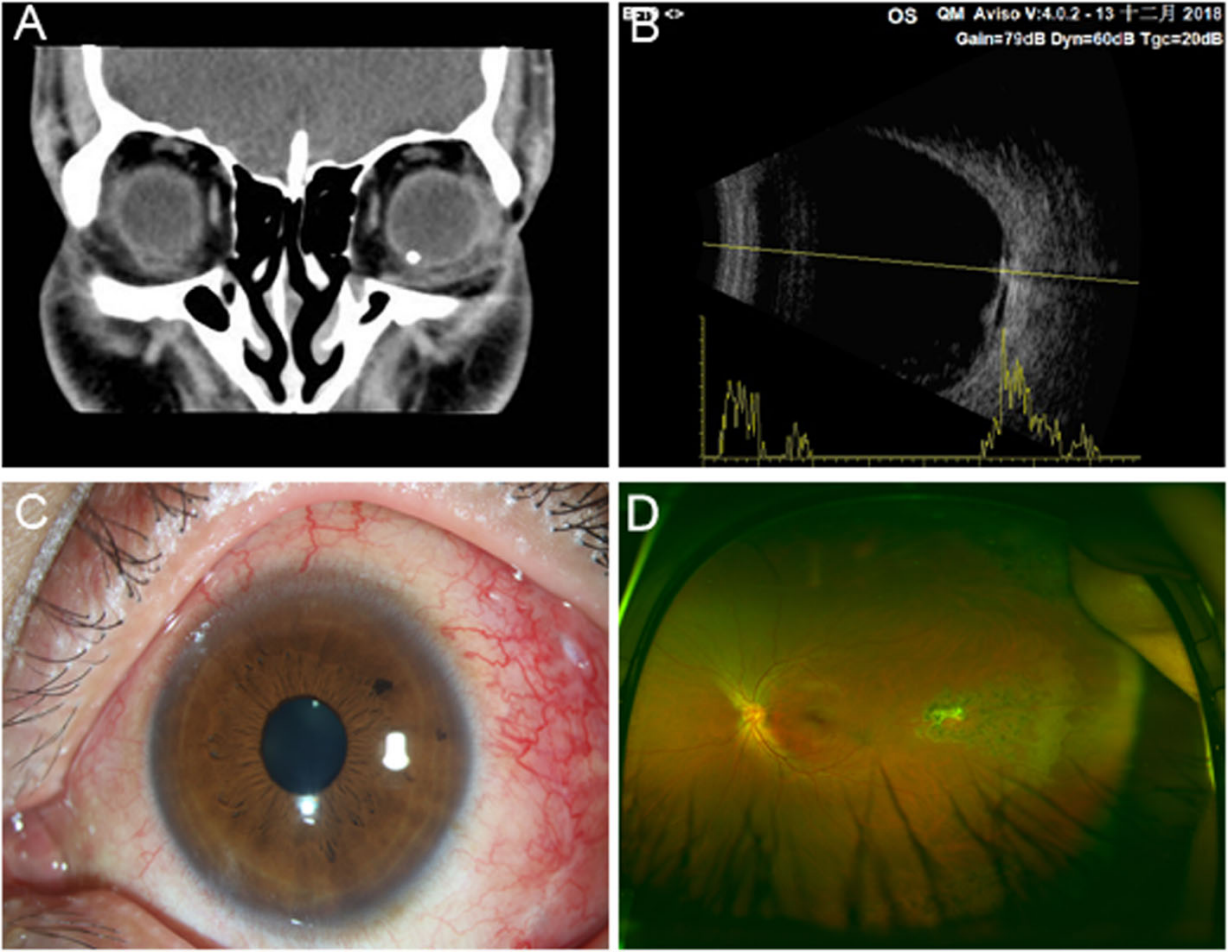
A patient with positive foreign body in the bulb of the left eye. (a) Preoperative coronal CT scan of the left eye. (b) Preoperative transverse CT scan of left eye. (c) Postoperative left anterior segment showed conjunctival congestion in the left eye, clear cornea, sensitive pupil response to light, transparent lens. (d) Left eye fundus photography after surgery showed vitreous fluid filling in left eye, retinal flattening, old laser spot in temporal macular area, White scar in the central laser spot
